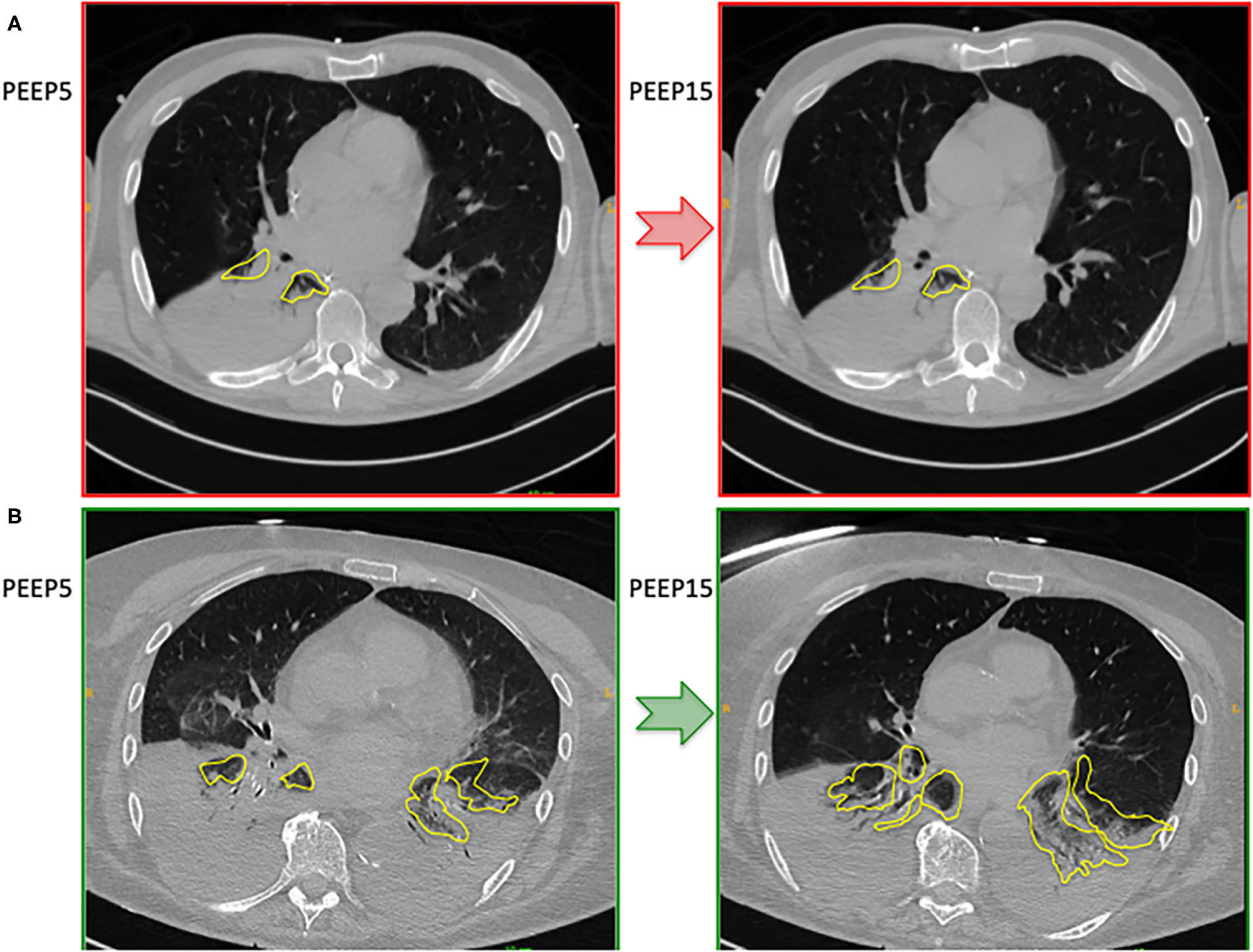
Representative cases representing CT images at 5 and 15 cmH_2_O of PEEP in a patient with poor alveolar recruitment (A) and good alveolar recruitment (B).
